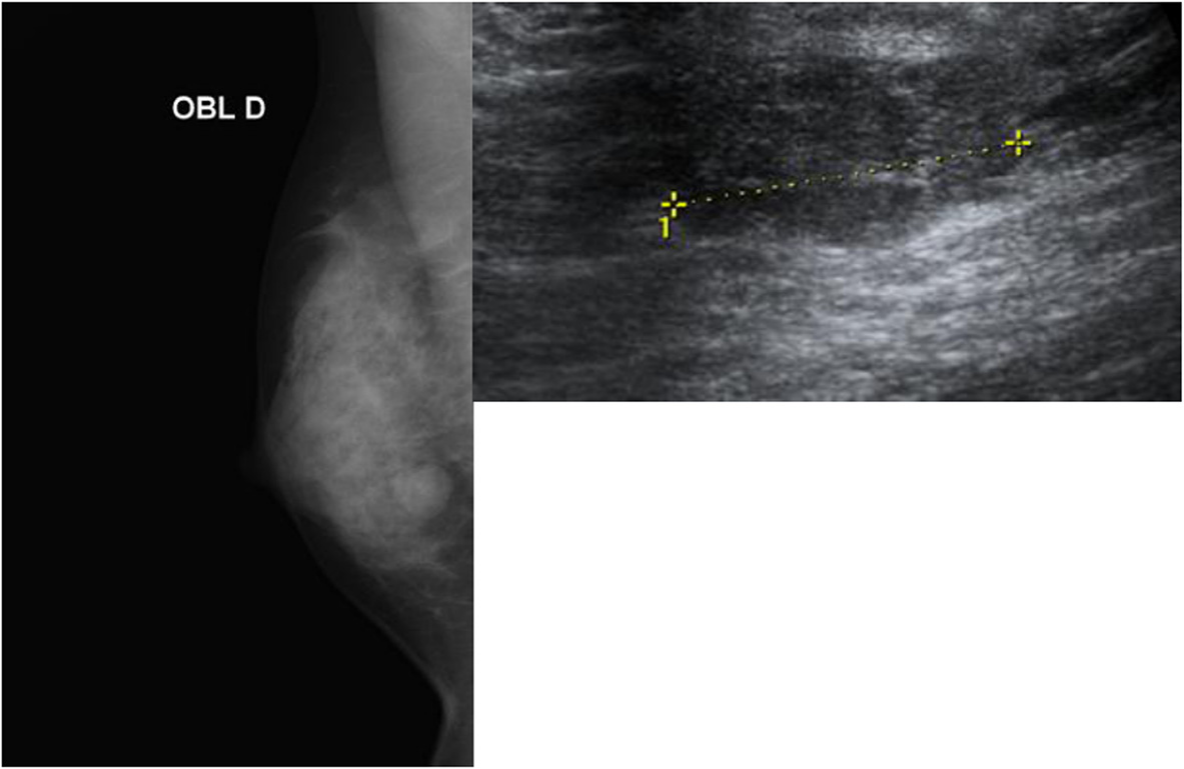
Mammography showing a round,well delineated opaque image in the lower outer quadrant of the right breast. Ultrasound showing a well delineated bilobed tissue lesion measuring 15 mm.
